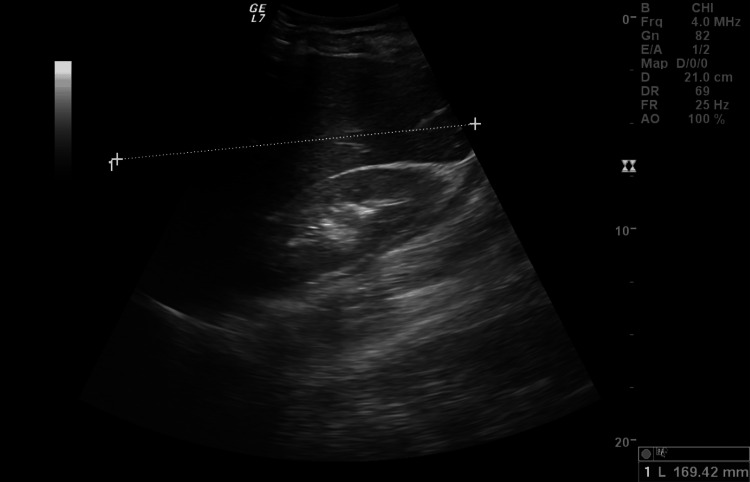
Hepatomegaly on abdominal ultrasound. Craniocaudal length: 169.42 mm
